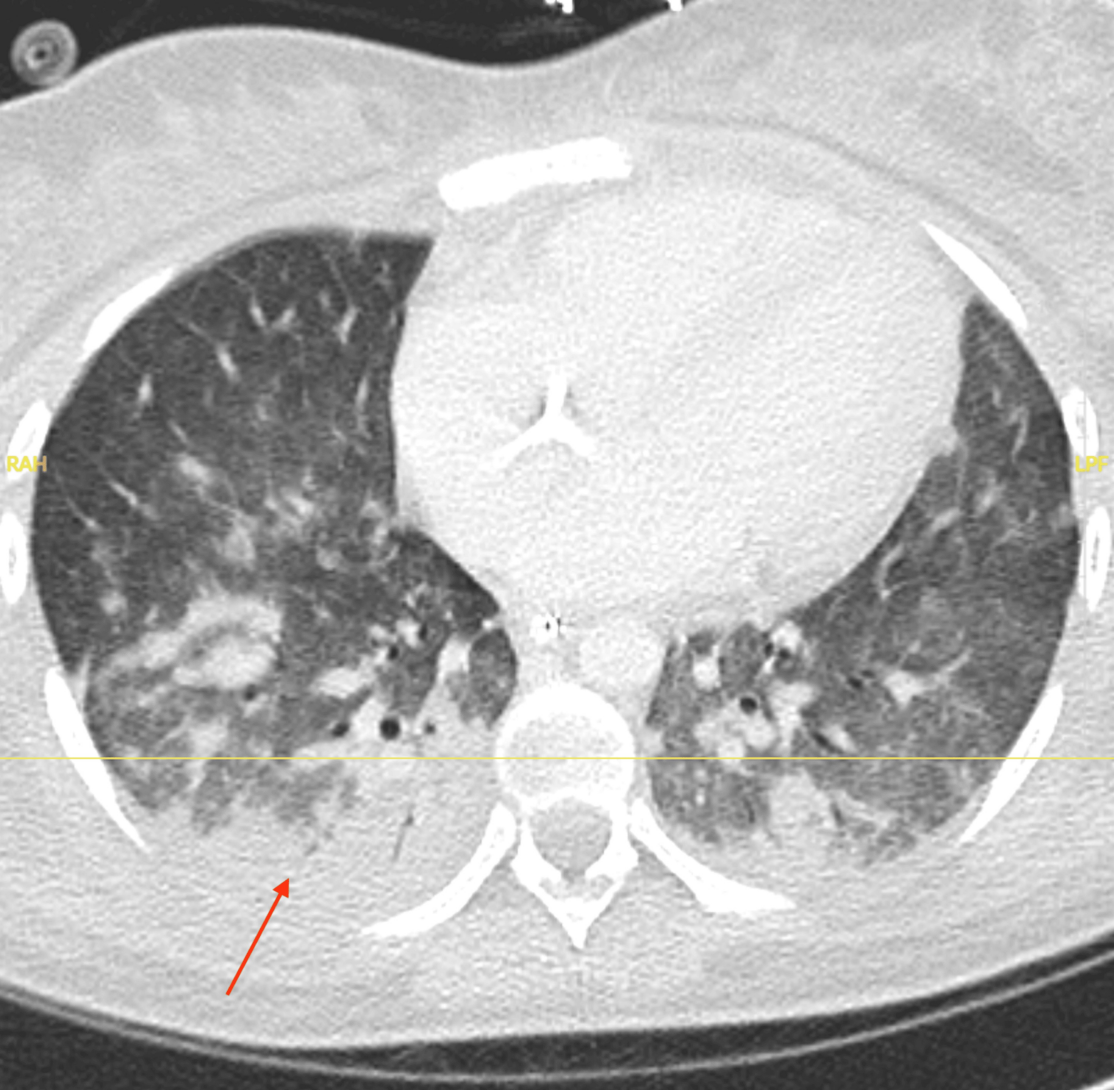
CT scan image of the thorax. The figure shows bilateral consolidation infiltrates in the lung bases. Innumerable ill-defined nodular ground-glass opacities are seen bilaterally in the lungs, likely representing atypical infiltrates. There is also underlying pulmonary venous congestion and mild interstitial edema. Extensive bibasal atelectasis is noted. Axial CT images are displayed in the standard anatomical orientation, with the patient's anterior (front) positioned toward the top of the image and the posterior (back) toward the bottom. CT was obtained on 02/21/2025.
